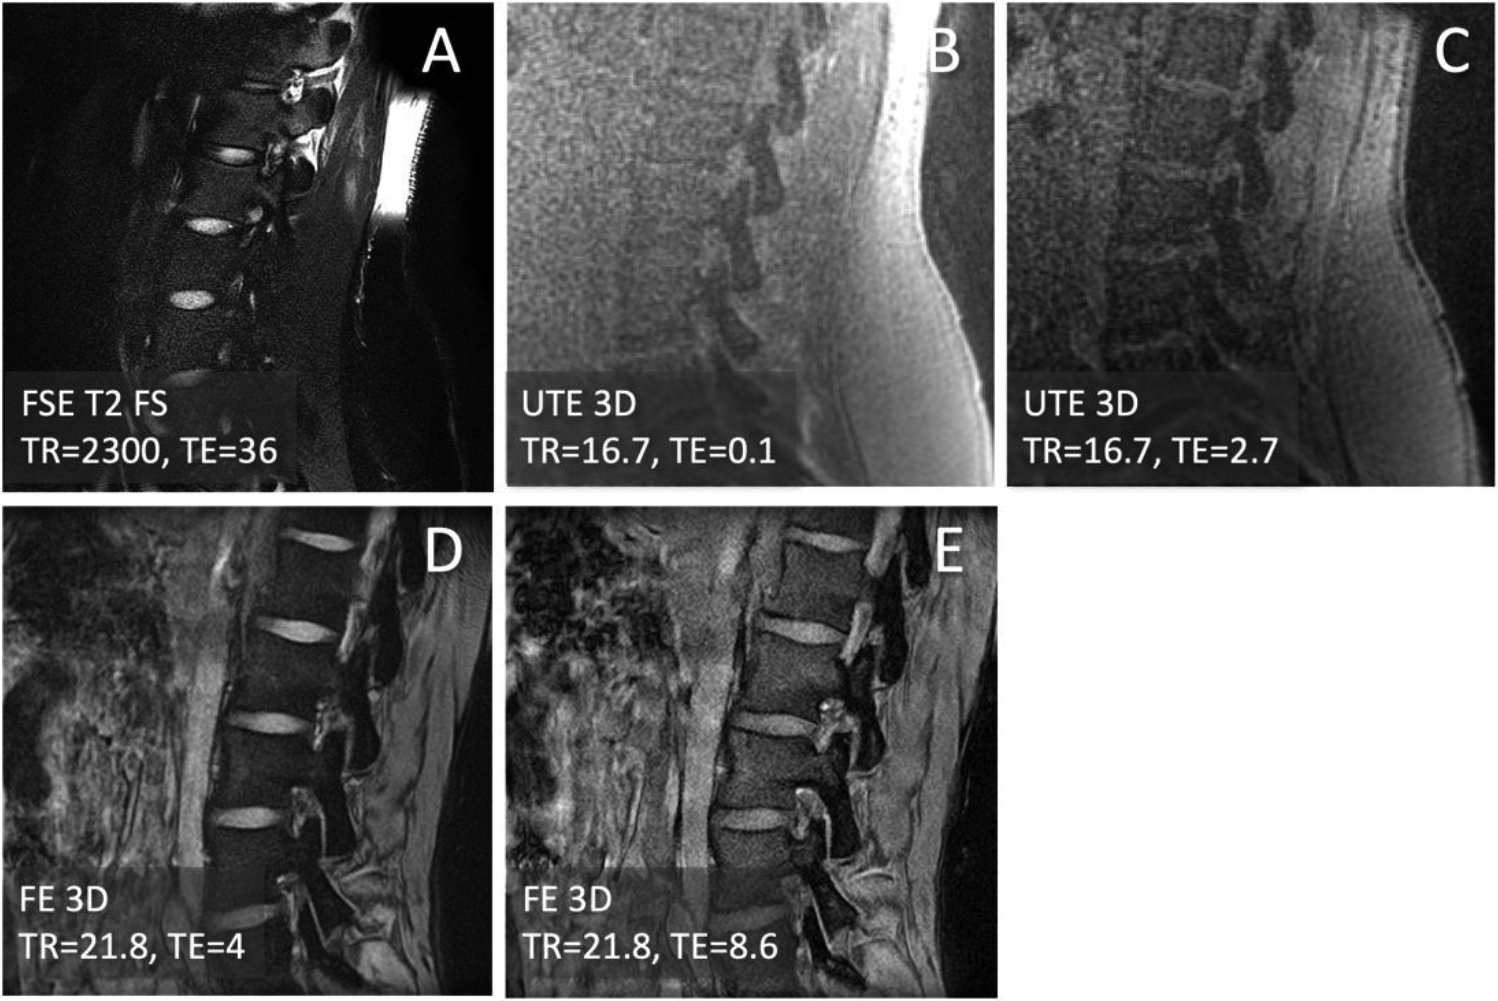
Raw MRI images of a lumbar spine acquired with (A) fast spin echo T2 weighted fat suppressed (FSE T2 FS), (B, C) 3D ultrashort echo time (UTE) at varying echo times (TE), and (D, E) 3D field echo (FE) at varying TE.
